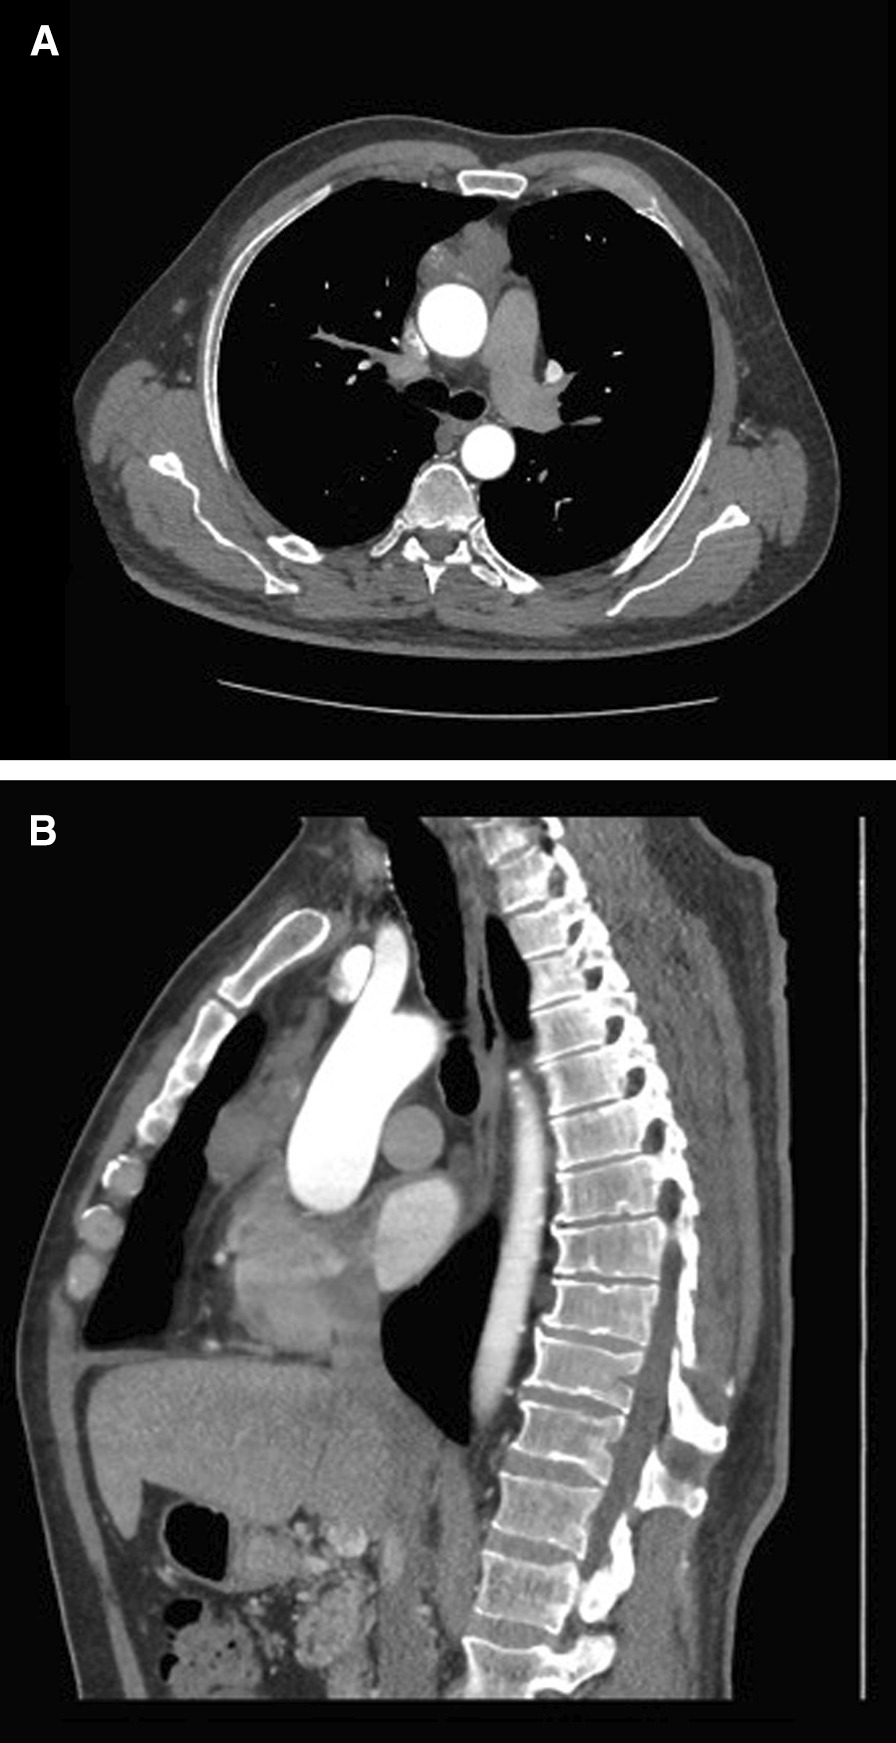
Axial (A) and Sagittal (B) representative CT images of the anterior mediastinal mass with abutment of the aorta and pericardium
